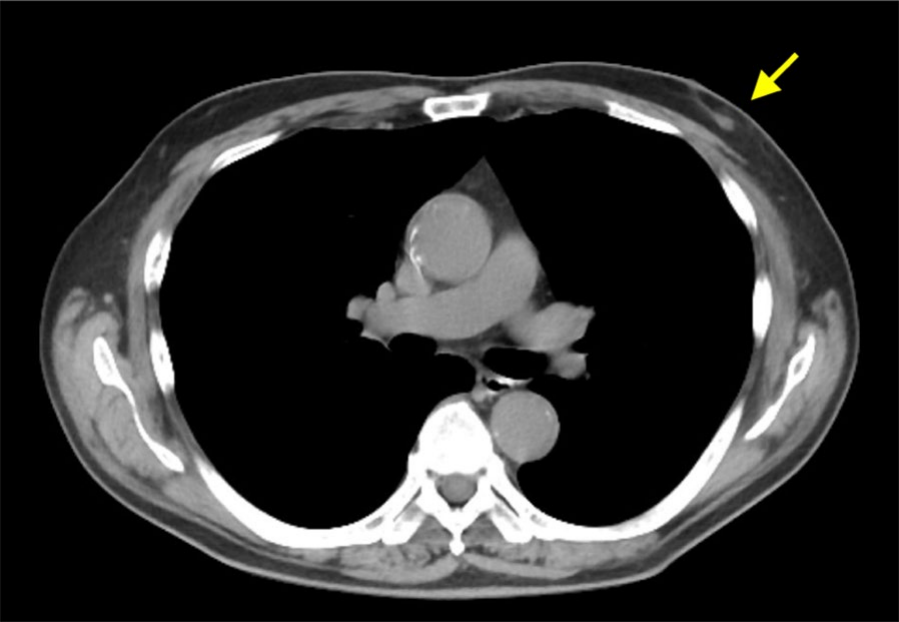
CT revealed a mass lesion with a linear shadow between the mass and nipple in the patient’s left breast
